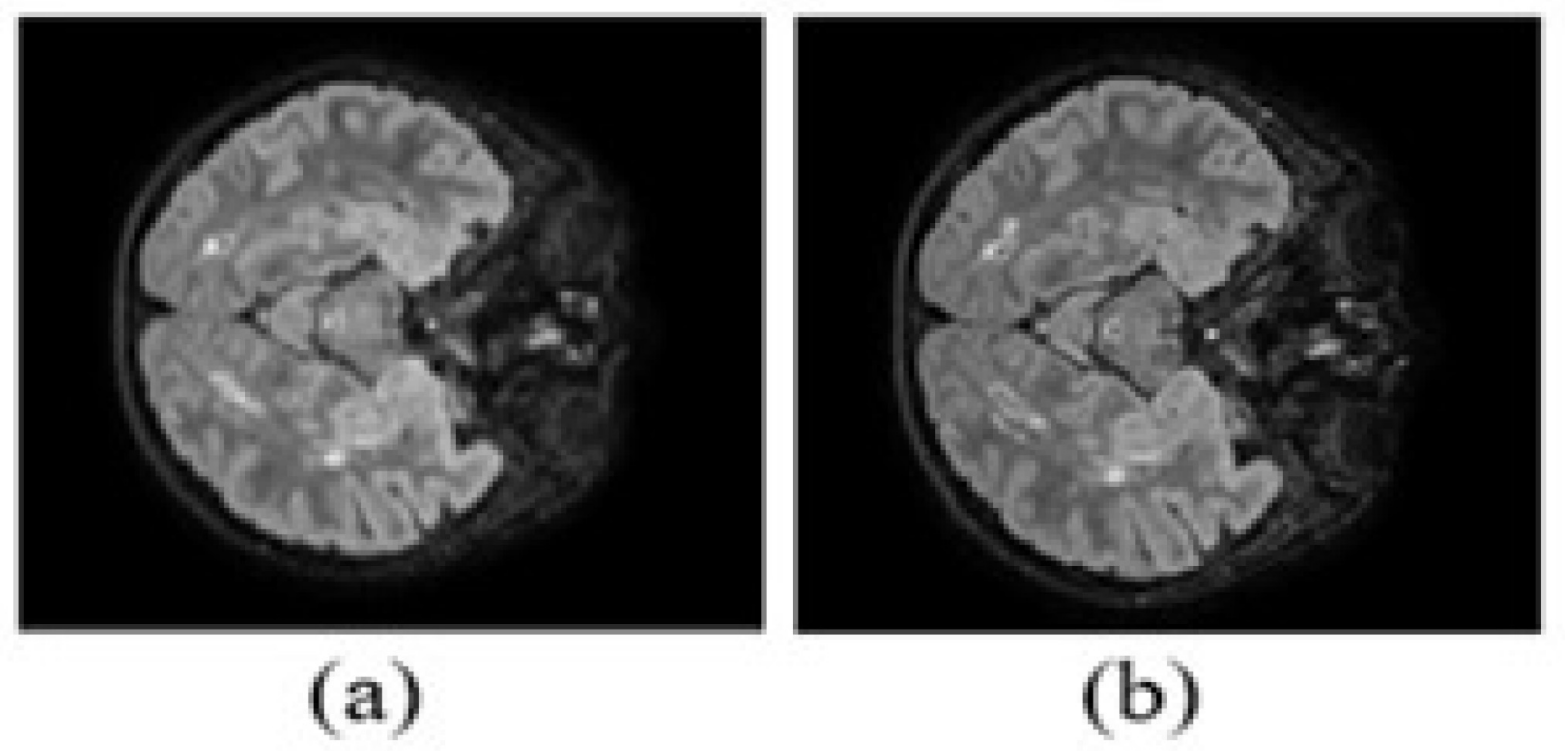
MICCAI-2021 dataset sample brain MRI in FLAIR modalities when slice number is 241 for the thirty-fifth patient in the dataset. (a) Time point-1 image. (b) Time point-2 image.
